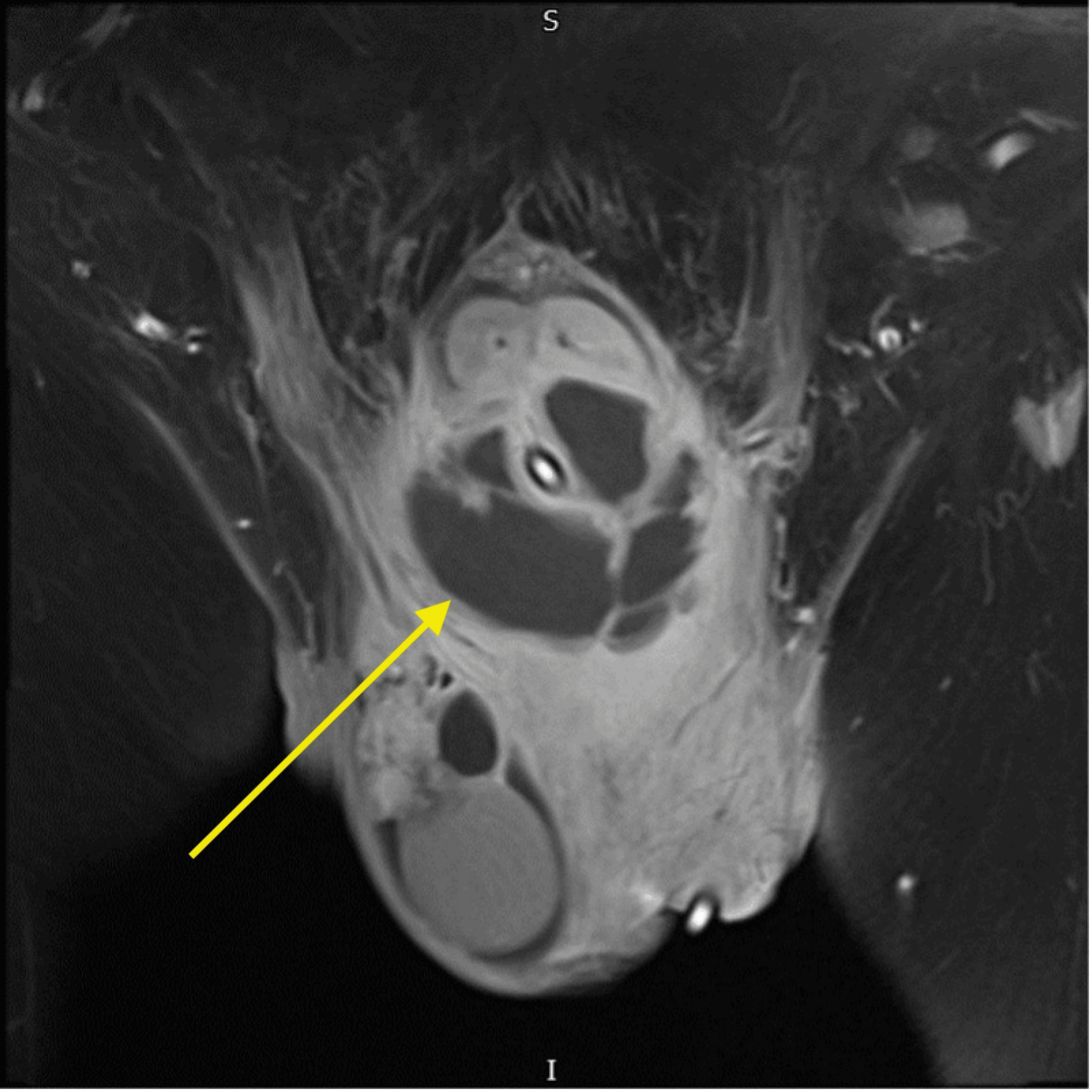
MRI T1 coronal image of the perineum. Yellow arrow highlighting the area of low attenuation, suggestive of an abscess.
